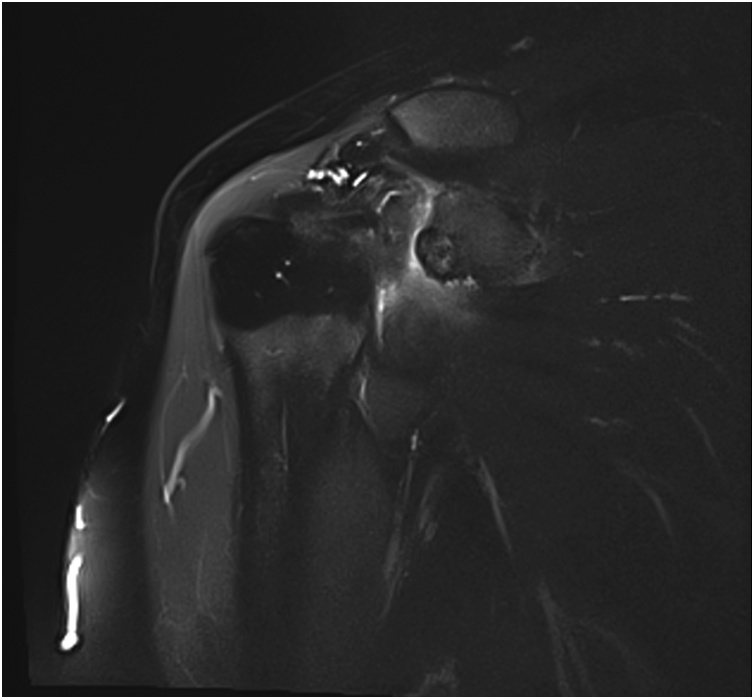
Contrast-enhanced MRI of the right shoulder.
